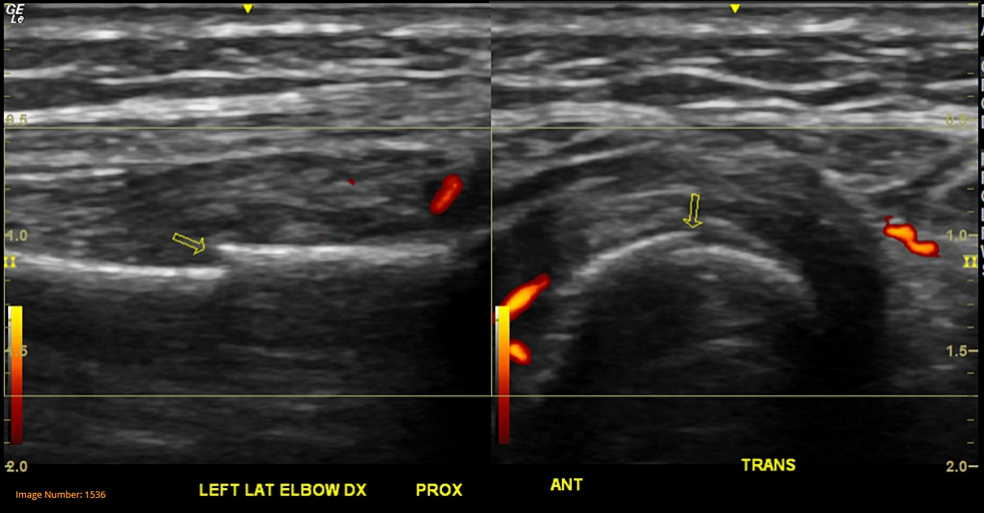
Forearm ultrasound showing blood flow near the site of the fracture Ultrasound image of the patient’s left forearm in longitudinal and transverse views. The figure shows arterial blood flow through the region with the fracture identified by the arrow. Doppler flow (shown in red) depicts no damage to surrounding structures.
